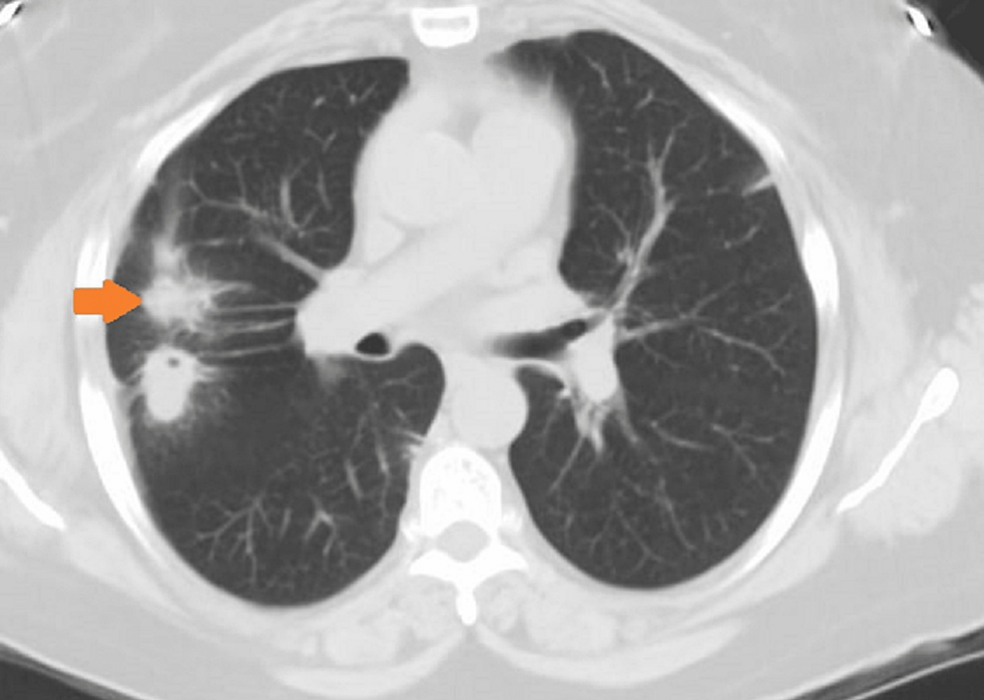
CT of the chest showing persistent right lung mass with possible abscess (arrow) and resolved left lung mass.
